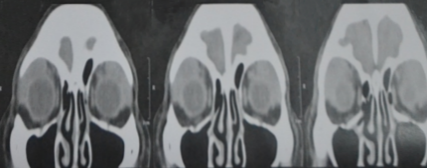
A coronal view of a plain orbital CT scan of the patient showing a homogenous mass molding around the globe and recti bilaterally
